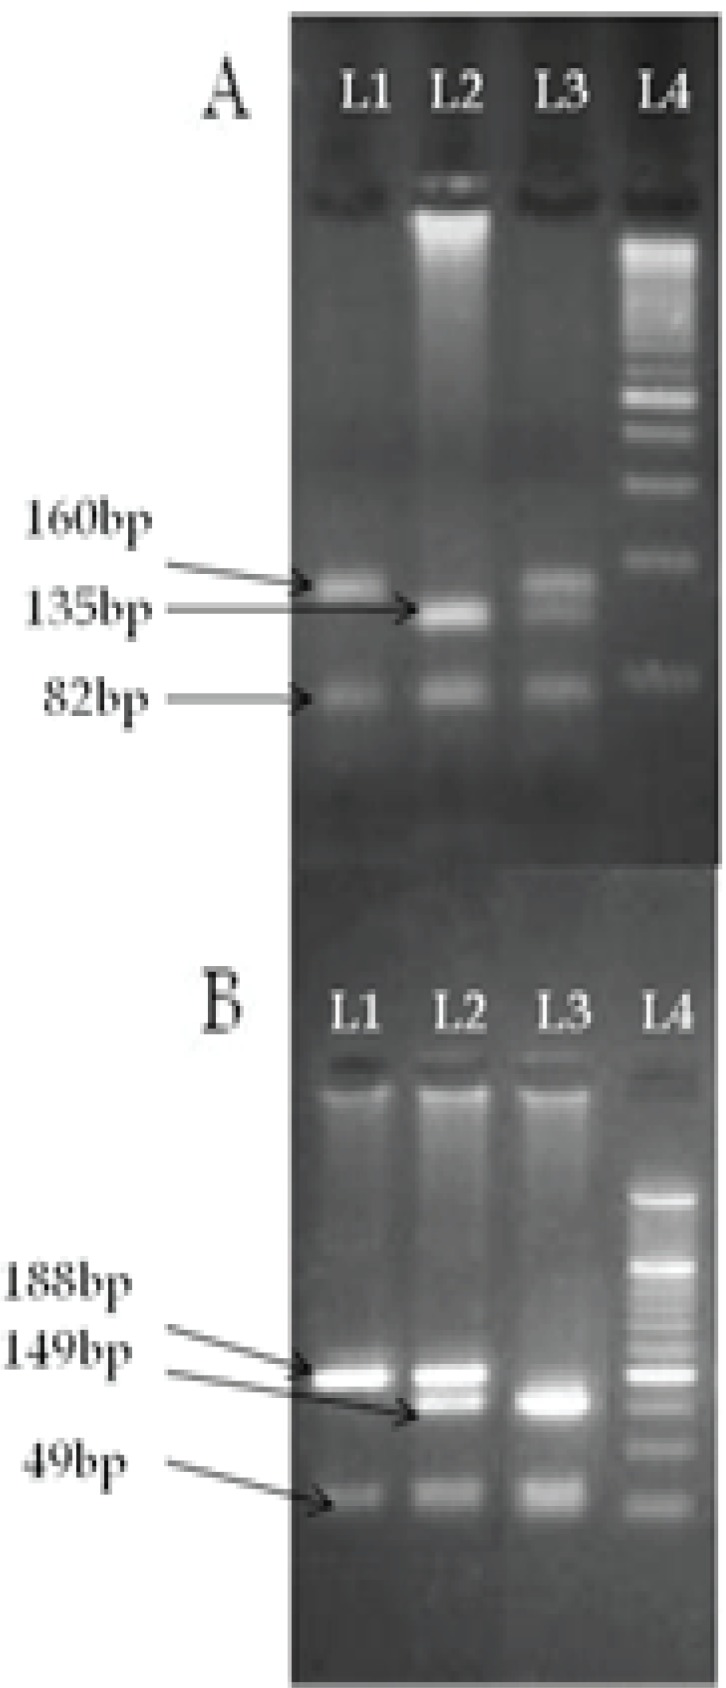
A: rs12979860 fragments. L1: TT genotype. L2: CC genotype. L3: CT genotype. L4: 100bp DNA ladder. B: rs8099917 fragments. L1: TT genotype. L2: TG genotype. L3: GG genotype. L4: 50bp DNA ladder
